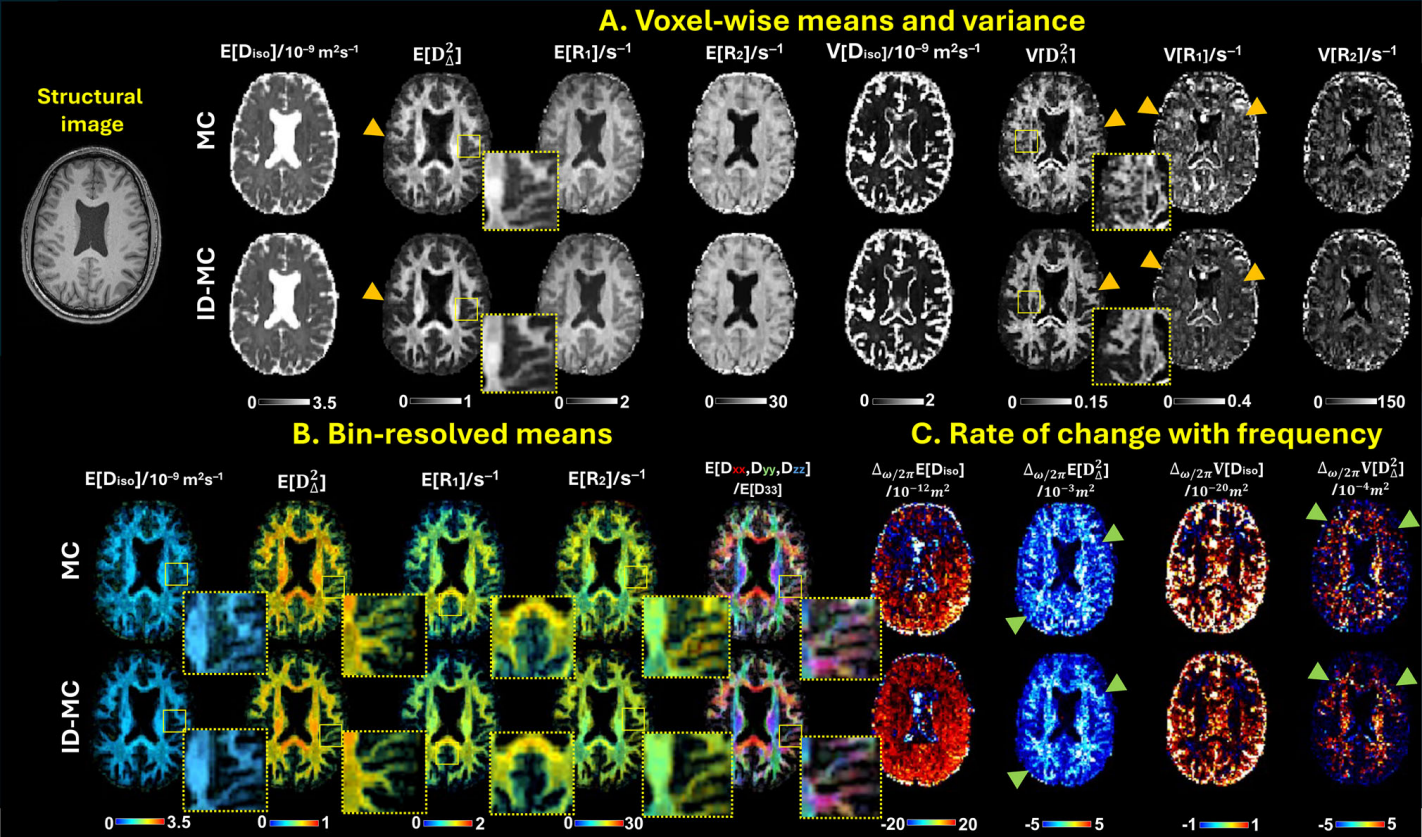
Qualitative comparison of MD‐MRI parameter maps generated by MC and ID‐MC methods. ID‐MC (with $N_{m}=1$) yields superior noise reduction (orange arrows) and clearer structural definition (zoomed images) across various parameters. Furthermore, the rate of change in parameter means and variances as a function of diffusion encoding frequency reveals not only clearer structural delineation but also a substantial reduction in apparent noise (green arrows).
